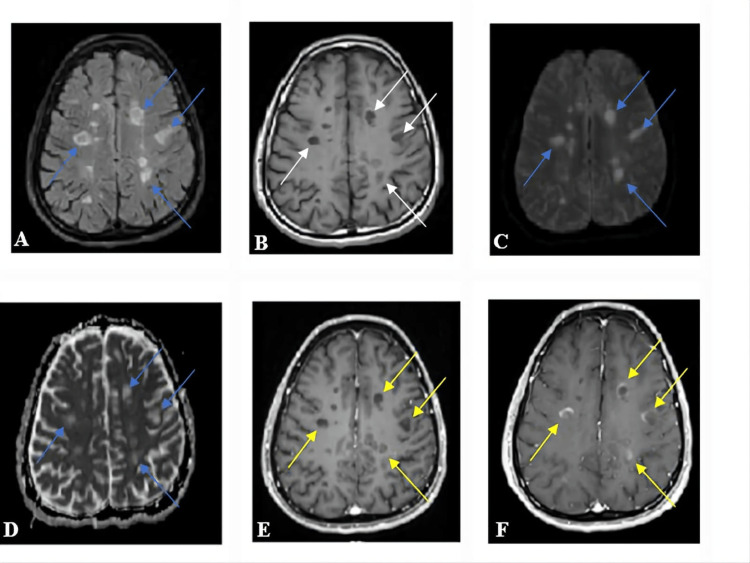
A 38-year-old male patient with active MS and brain periventricular multiple demyelinating plaques. (A) Axial FLAIR showed high signal intensity (blue arrows). (B) Same lesions in axial T1-weighted images were hypointense (white arrows). (C) Axial DWI and (D) the ADC map were hyperintense (blue arrows). (E) Axial MPRAGE in the early post-contrast enhancement with no contrast uptake in any of the lesions (yellow arrows). (F) Delayed phase enhancement; the lesions were fully enhanced (yellow arrows). MS, multiple sclerosis; FLAIR, fluid attenuation inversion recovery; DWI, diffusion-weighted image; ADC, apparent diffusion coefficient; MPRAGE, magnetization-prepared rapid acquisition gradient echo
